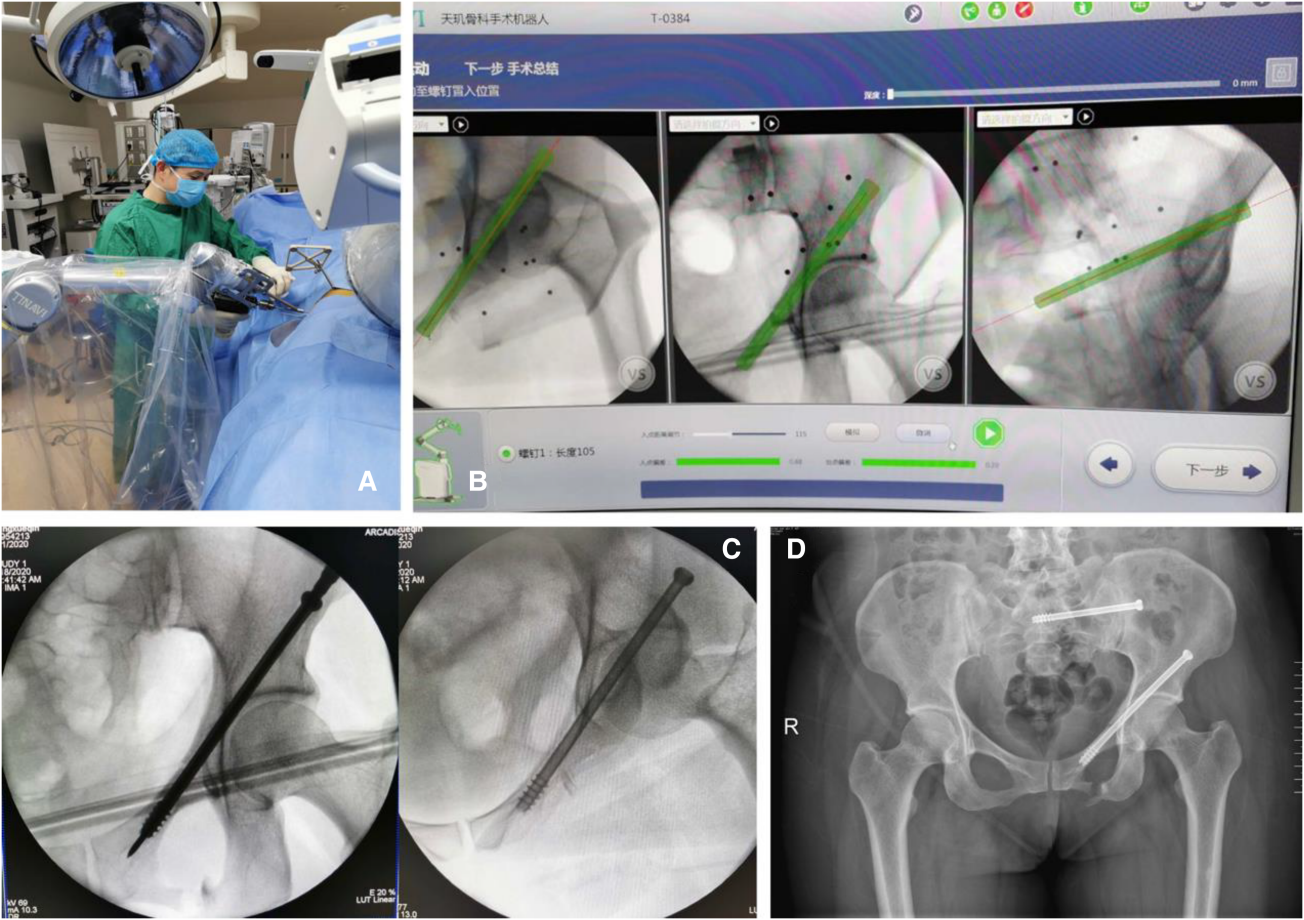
(A) Intraoperative manipulation of the surgical robot; (B) computer-generated planning image during the surgery; (C) guide wire for the percutaneous insertion of hollow screws guided by computer planning and the surgical robot; (D) postoperative x-ray photo.
